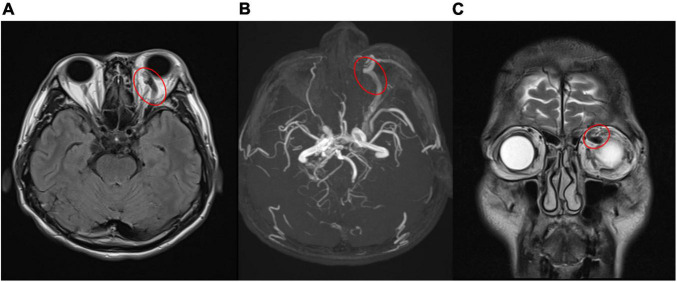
(A,B) Engorged left superior ophthalmic vein (red circle) in magnetic resonance imaging (A), magnetic resonance angiography (B), which confirmed carotid- cavernous sinus fistula. [(A) T2-weighted-Fluid-Attenuated Inversion Recovery, transverse view; (B) magnetic resonance angiography]. (C) Coronal view of magnetic resonance imaging revealed prominent engorged superior ophthalmic vein (red circle) [T2- Turbo spin echo (TSE) imaging, coronal view].
